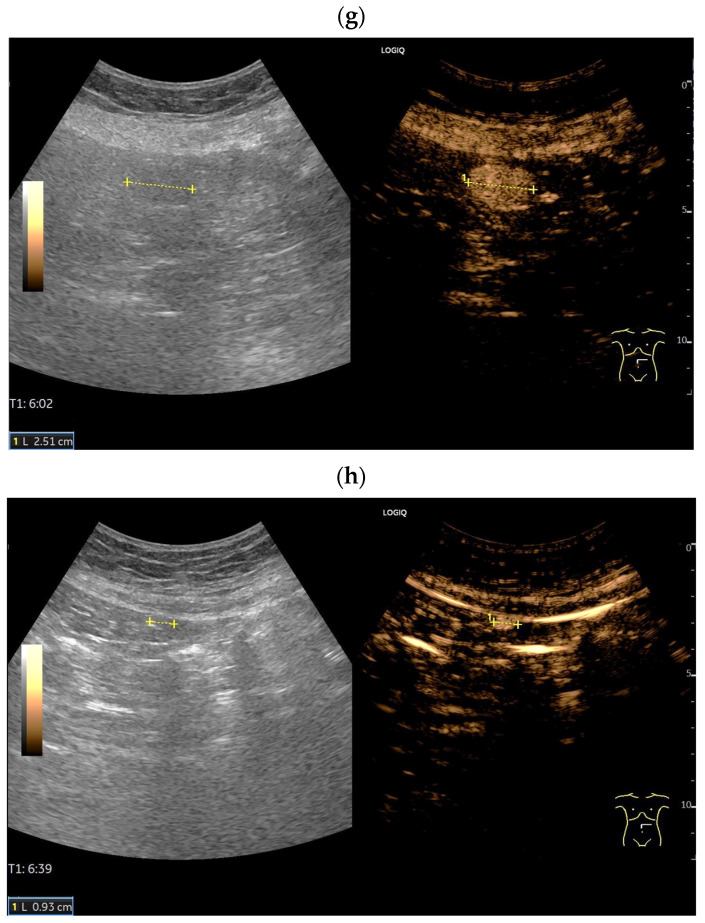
Splenosis-intrahepatic and intraperitoneal. US was performed on a patient with deep vein thrombosis to rule out paraneoplastic syndrome. The patient had undergone a splenectomy more than 10 years ago, following a motorcycle accident. In the liver, two well-defined oval and round hypoechoic lesions (arrows) were observed subcapsular in the right lobe of the liver, with moderate hepatic steatosis (a). Multiple round and oval lesions were also visible in the abdomen (arrow) (b). Contrast-enhanced ultrasound (CEUS) with 1.2 mL of SonoVue showed homogeneous hyperenhancement of the liver lesions (between the markers), which persisted into the late phase. This was observed at 3:52 min (c,d) and still at 7:39 min (e). The multiple round lesions in the abdomen also showed prolonged enhancement (f,g), and lesions were revealed that had not been previously visible on just B-mode ultrasound alone (h).
